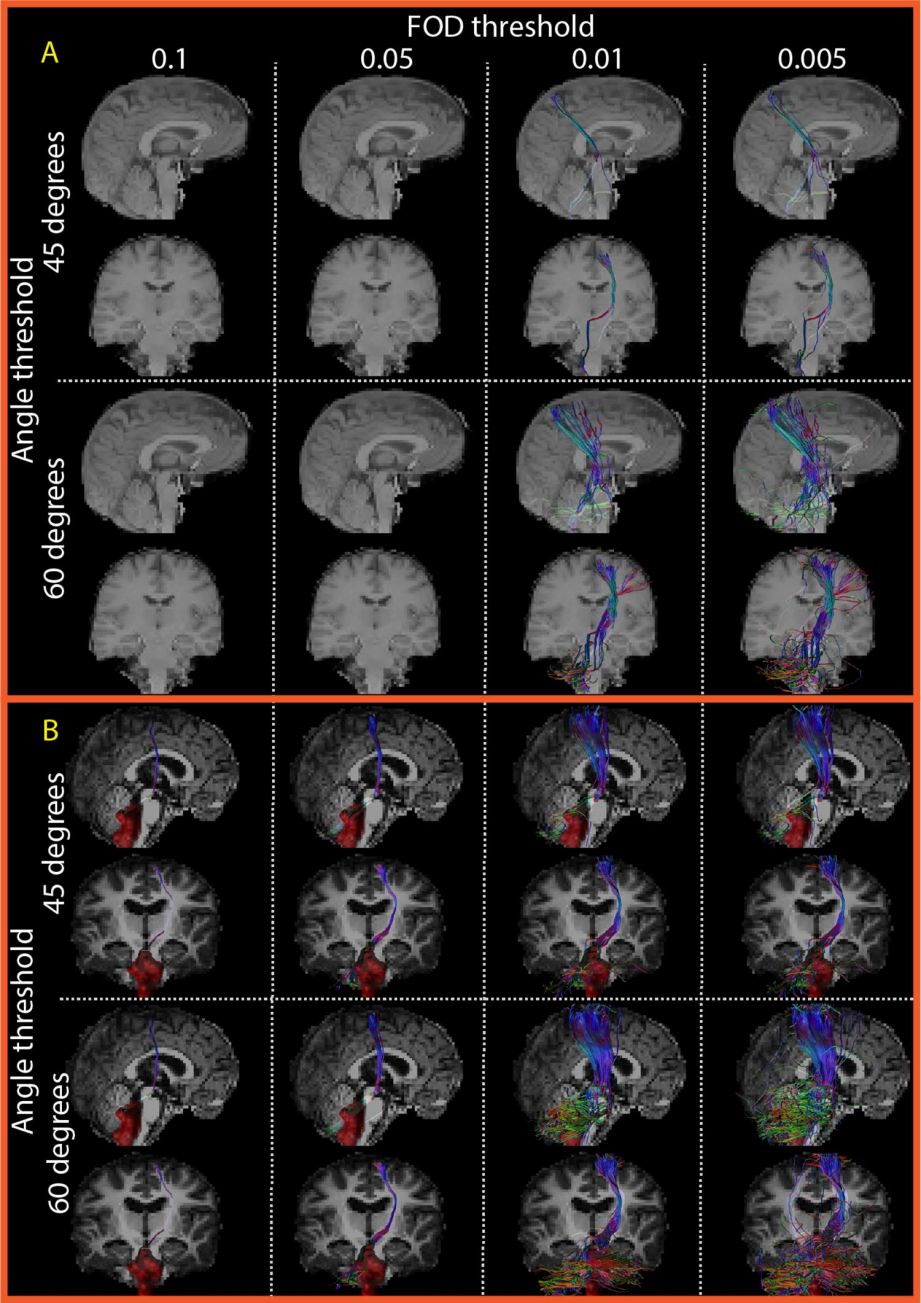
Example of eight fiber tractography parameter combinations for one side dentato-rubro-thalamic tract. All panels show the dentato-rubro-thalamic tract (DRTT) crossing from the dentate nucleus to the contralateral hemisphere. The upper panel displays data from a healthy volunteer, and the lower panel shows preoperative results for an example patient. More liberal settings increased visual coherence of streamlines but introduced false positives, as seen with an FOD threshold of 0.005 and an angle threshold of 60°. Parameter combinations were presented in a randomized order and blinded for the panel. A different T1-weighted contrast was used for healthy volunteers and patients
